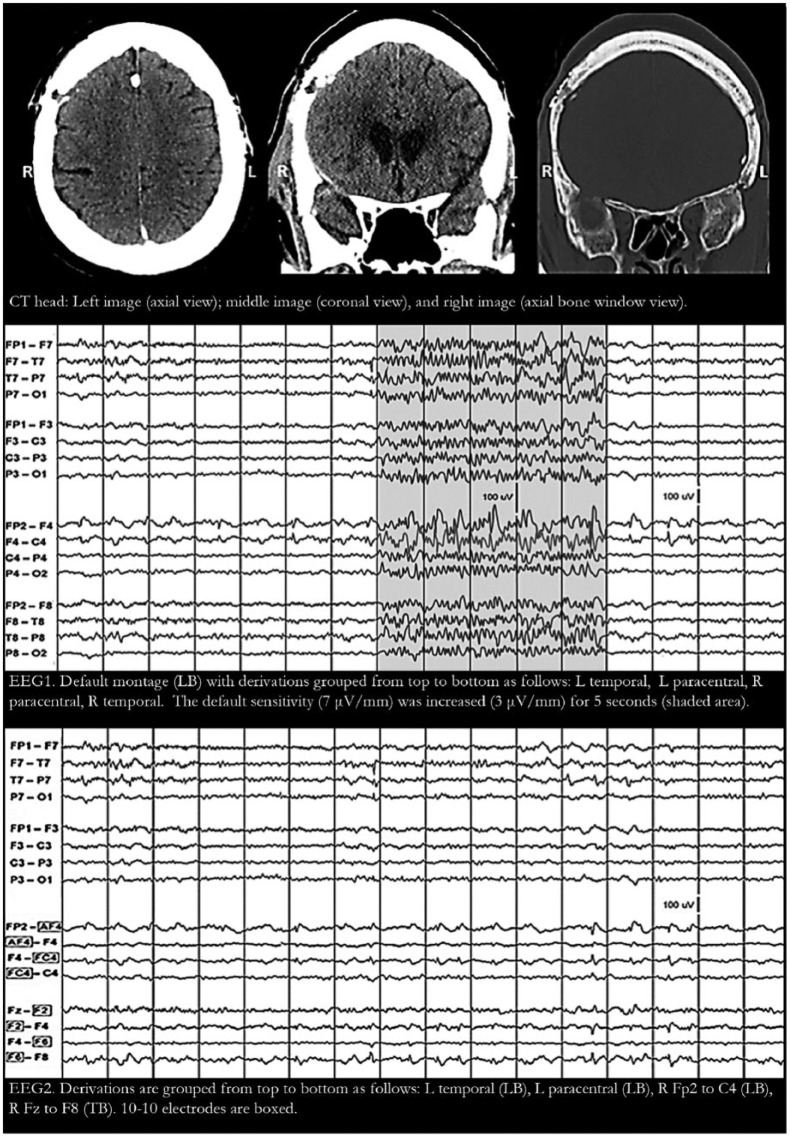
Patient 2 has residual skull holes in the frontal area from a meningioma resection surgery (CT [computed tomography] head). Electroencephalogram (EEG) showed epileptiform spikes in T3 and F7 (both with a clear-cut physiological field) and phase reversals in FP2-F4 and F4-C4 consistent with an F4 electrode artifact (EEG1). Increasing display sensitivity showed an occasional hint of a physiological field but this was not enough to rule out electrode artifact (EEG1 shaded area). Adding 10-10 electrodes (AF4, FC4, F2, and F6) and extending the montage showed that the F4 potentials have a physiological field (EEG2). For all EEG tracings above: filters at 1-Hz high-pass, 70-Hz low-pass, and 60-Hz notch; 1 second/division; and 100-µV voltage scale shown in tracing. L, left; R, right; Ref, reference; LB, longitudinal bipolar; TB, transverse bipolar; LR, longitudinal referential; TR, transverse referential.
